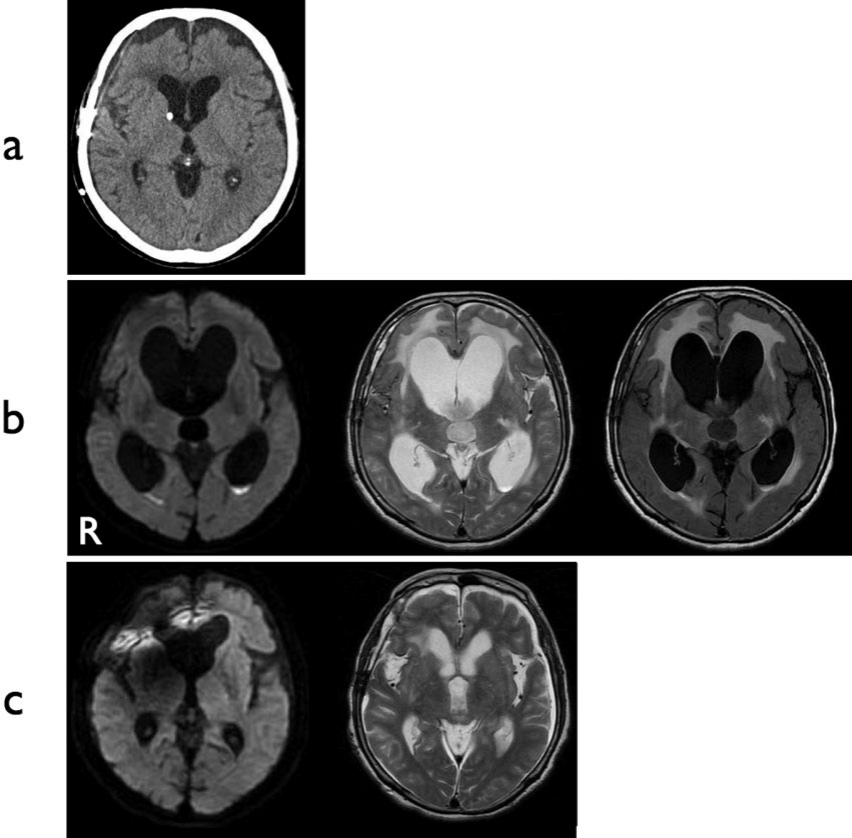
Case 1. (a) CT after the VP shunt had been placed and 2 months before this admission. (b) MRI on admission. Diffusion-weighted imaging (DWI) shows abnormal hyperintensities in the trigones of both lateral ventricles, suggesting debris (left panel). T_2_-weighted imaging (central panel) and fluid-attenuated inversion recovery imaging (right panel) show abnormal periventricular intensities. (c) Postoperative MRI. DWI shows disappearance of hyperintense lesion in the ventricles. Hydrocephalus has resolved. A metallic artifact from the shunt system is present in the right frontal region. VP, ventriculoperitoneal.
